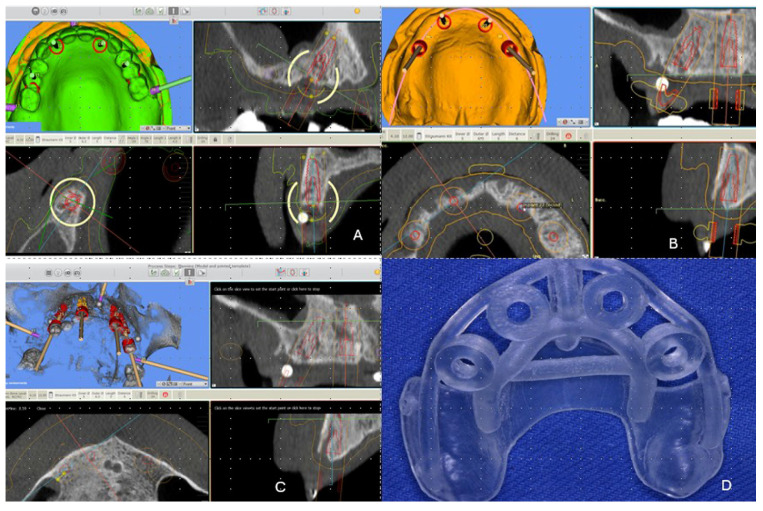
(A,B) Presurgical digital planning by superimposition of the STL files of the initial cast, the wax-up, and the CBCT. (C) Digital design of the surgical guide, (D) The printed surgical guide.
